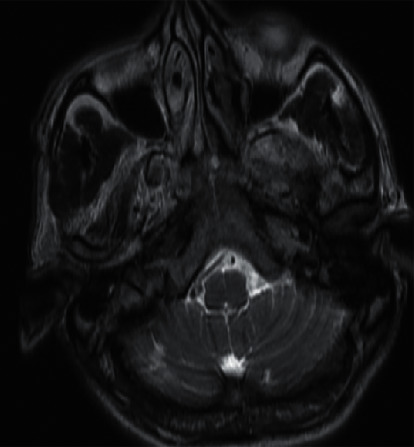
Axial view of MRI brain showing the right cerebellar infarct.
